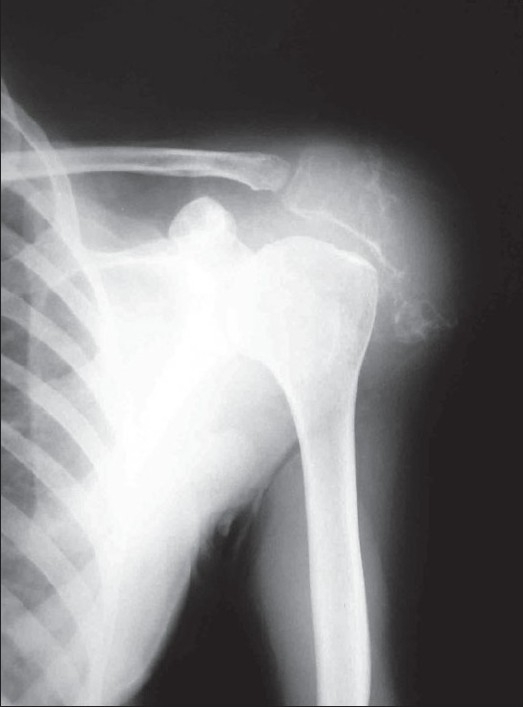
X-ray shows a lytic lesion with a soap bubble appearance in the acromion of the right scapula, with destruction of the periosteum and a prominent overlying soft tissue shadow
